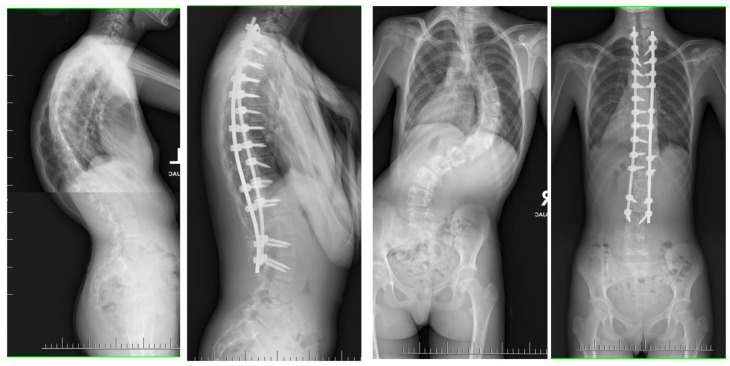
Radiographs obtained before and after the final follow-up examination revealed a 13-year-old female patient presenting with severe adolescent idiopathic scoliosis. The patient underwent treatment involving multi-level Ponte osteotomies followed by posterior spinal fusion in a single-stage surgical procedure.
